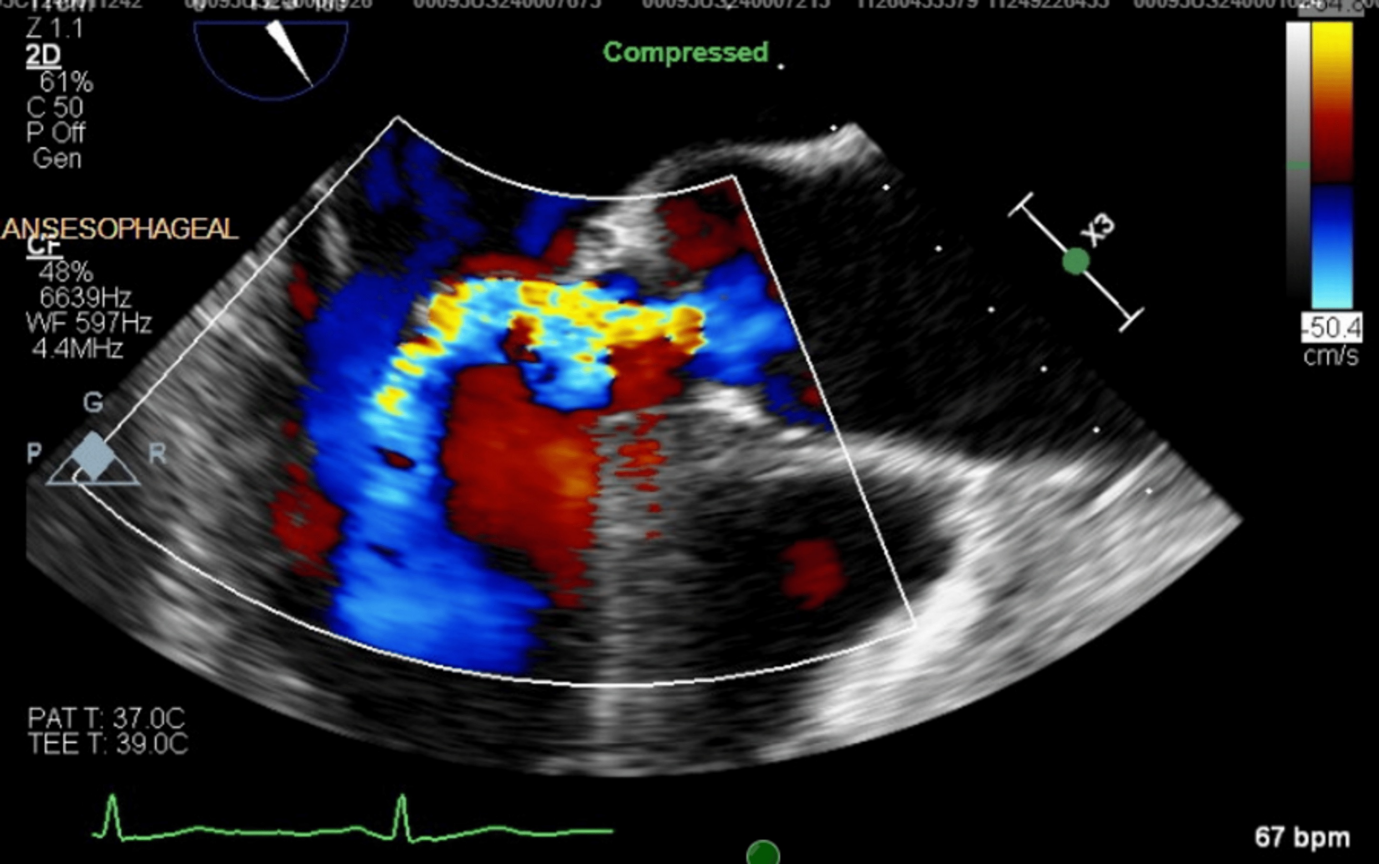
TEE color Doppler view showing aortic regurgitation TEE, transesophageal echocardiogram
